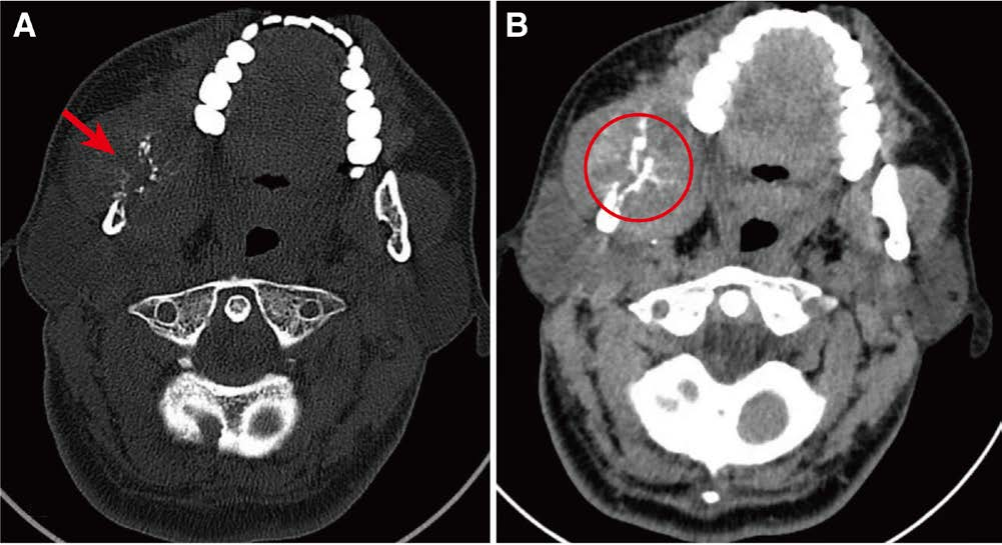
Computed tomography scans. (A) The bone window showed bone destruction of right mandible (red arrow). (B) The soft tissue window showed a soft tissue mass in the right mandible with radiolucent high density and distended growth with blurred margins, measuring approximately 4.8 cm × 3.8 cm × 4.9 cm at the largest level (red circle).
